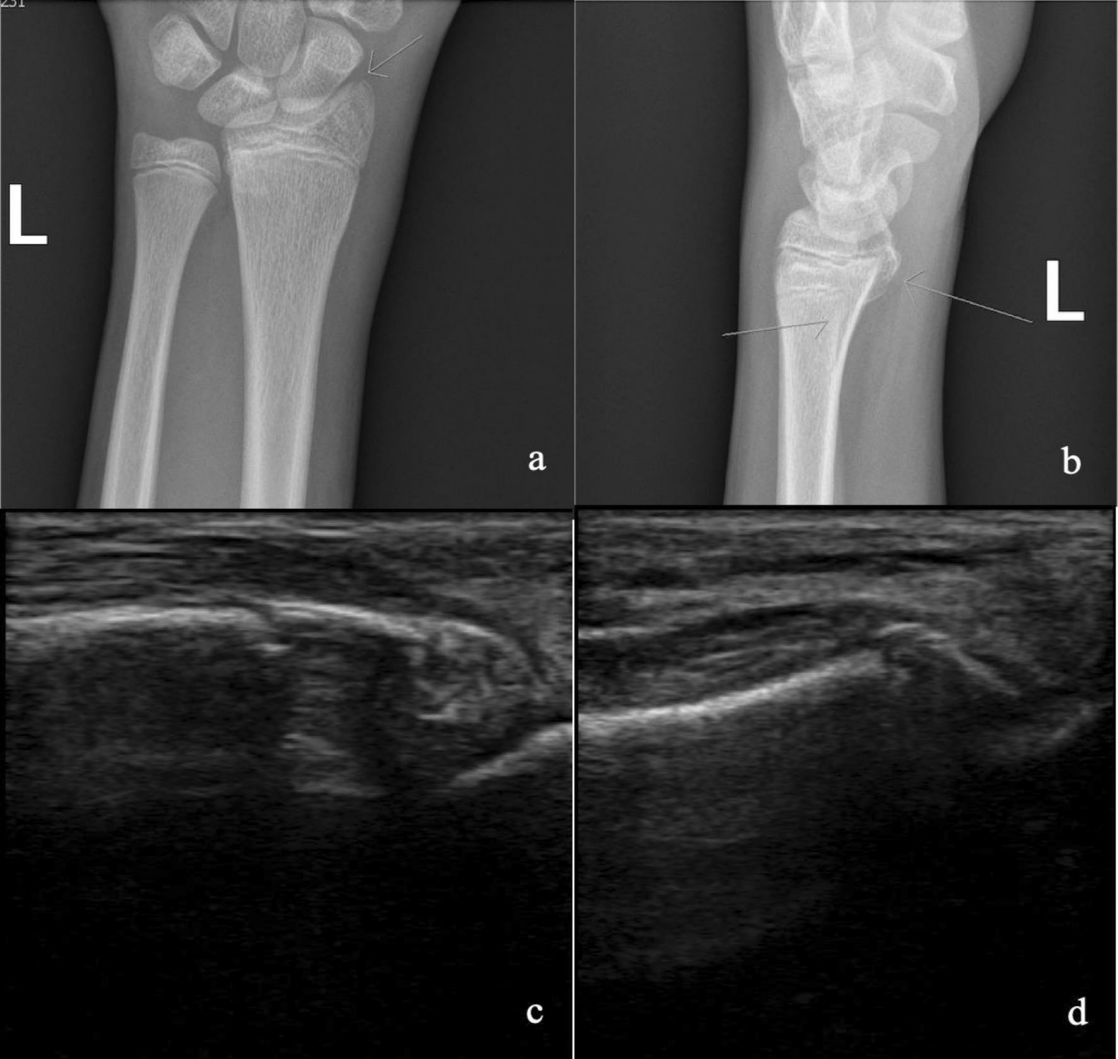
AP (a) and lateral (b) radiographs of a false negative US case, found to be a near anatomical, non-displaced Salter Harris 2 fracture, compared to radial (c) and volar (d) views of the corresponding area on 2D US. In hindsight there is a disruption in the physis cortex visible on 2DUS, though this was not appreciated at the time of the blinded expert read. This highlights the difficulty identifying a fracture when it is non-displaced and involves an open physis, which is already a discontinuity in cortex.
